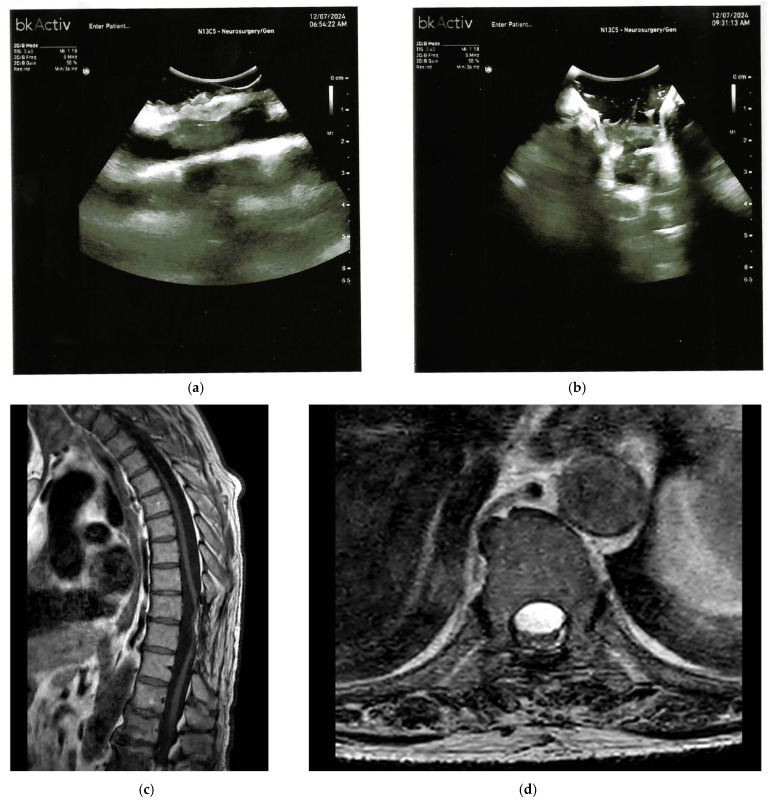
(a,b) Intraoperative B-mode ultrasound before release shows hyperechoic adhesions bridging dorsal dura and cord (arrow in (a) indicates adhesions) with near-complete effacement of the dorsal subarachnoid space and reduced cord excursion (labeled ‘effaced space’ in (b)). The hyperechoic adhesions on IOUS (a,b) correspond to the focal dorsal CSF loculation and anterior displacement on MRI (c,d). (c) Sagittal T2-weighted MRI demonstrates focal dorsal CSF loculation with anterior displacement of the thoracic cord (“scalpel sign”; arrow indicates displacement). (d) Axial T2-weighted MRI shows a crescentic dorsal subarachnoid collection compressing and anteriorly displacing the cord without a solid intradural mass findings consistent with tethering due to an arachnoid band/web (labeled ‘arachnoid band’).
